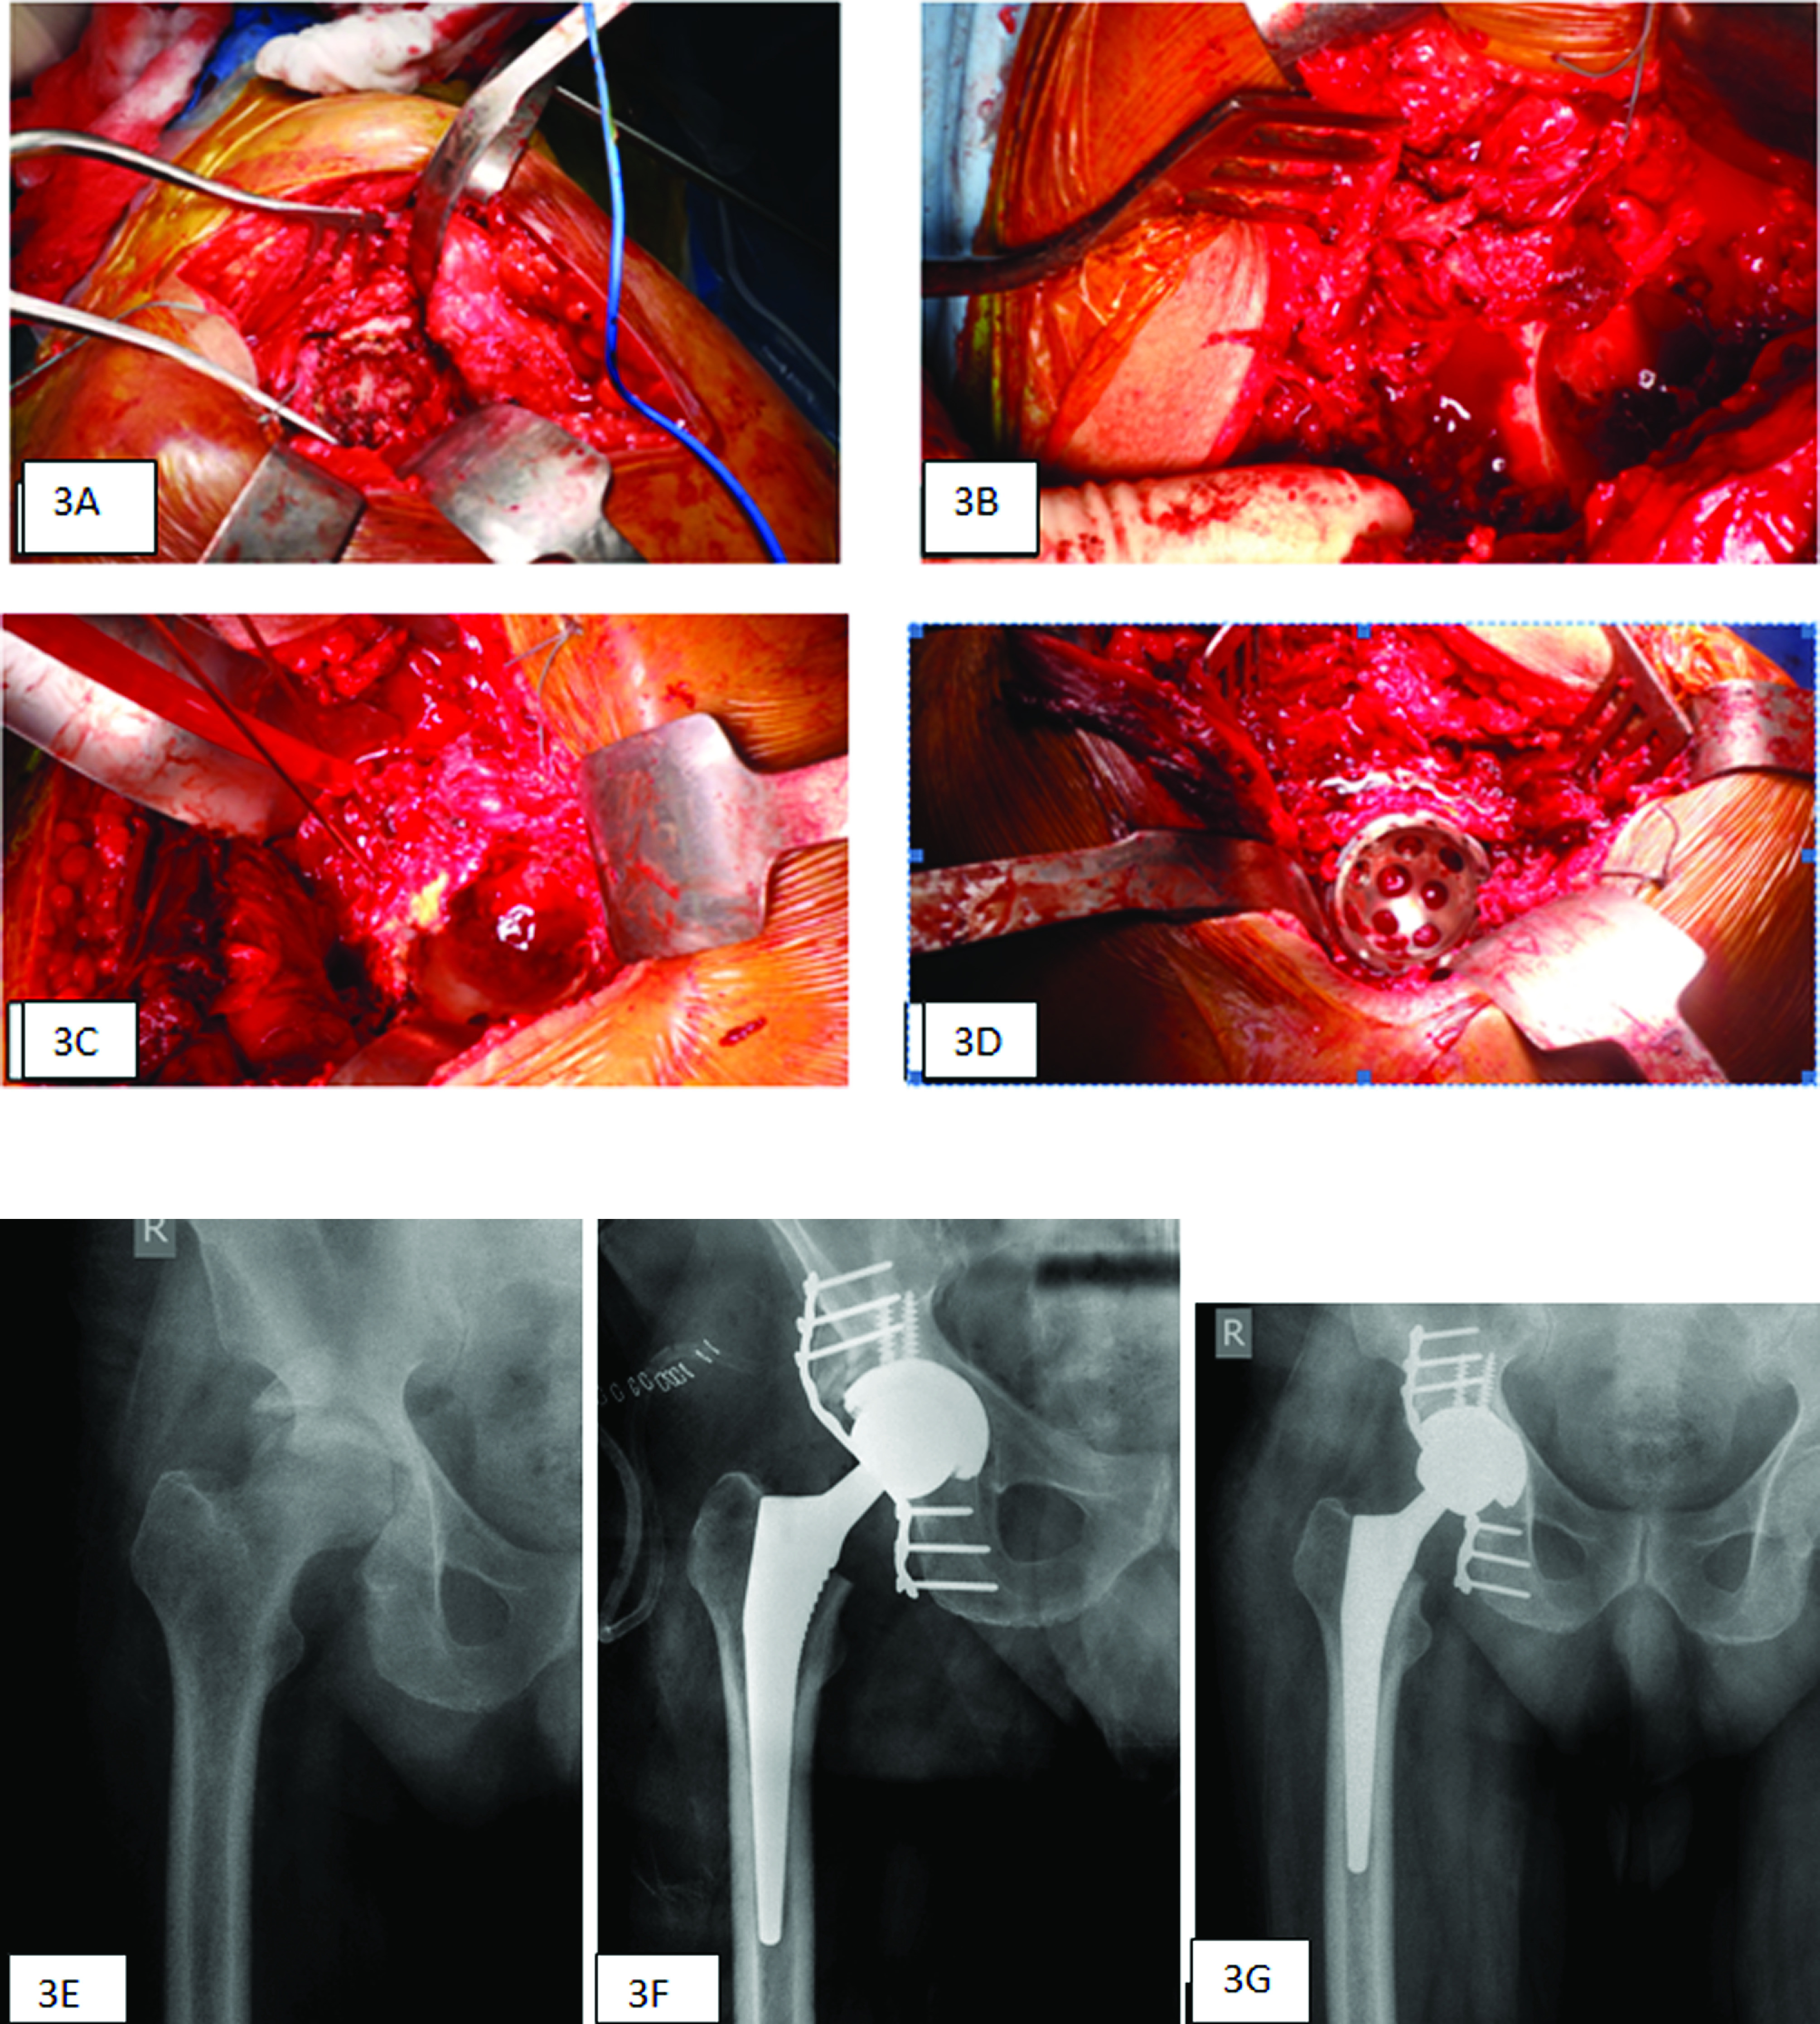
(A) 360° view of a neglected acetabular fracture and analysing the defect intra-operatively. (B) Posterior wall defect. (C) Provisional fixation of the defect with K-wires. (D) Final fixation with plate and cup. (E) Radiograph showing a posterosuperior wall acetabulum fracture. (F) Immediate post-operative radiograph showing fracture reduction and fixation with a plate. (G) Radiograph at 5-years follow-up.
